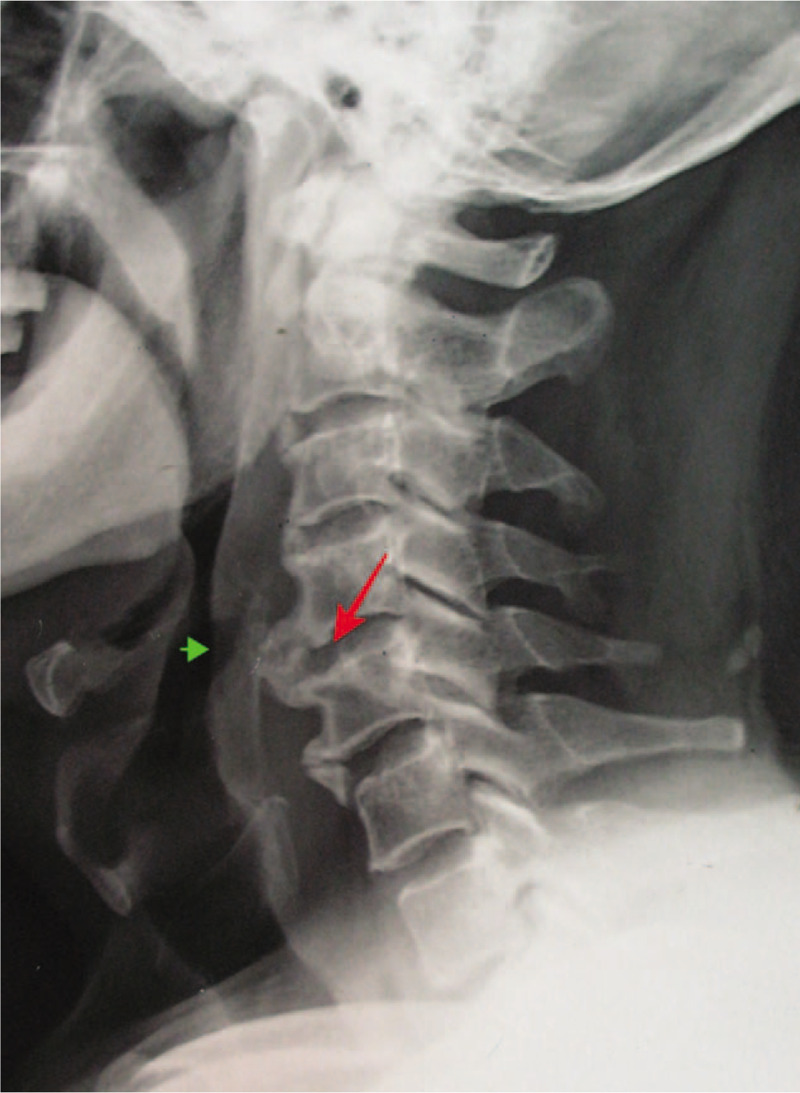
Lateral cervical spine radiograph showed a large flowing hyperostosis extended from the anterior aspect of C2 to C6, marked at the C4/C5 vertebrae (arrow). There was also a small niche shadow (arrowhead) on the posterior wall of the pharynx.
